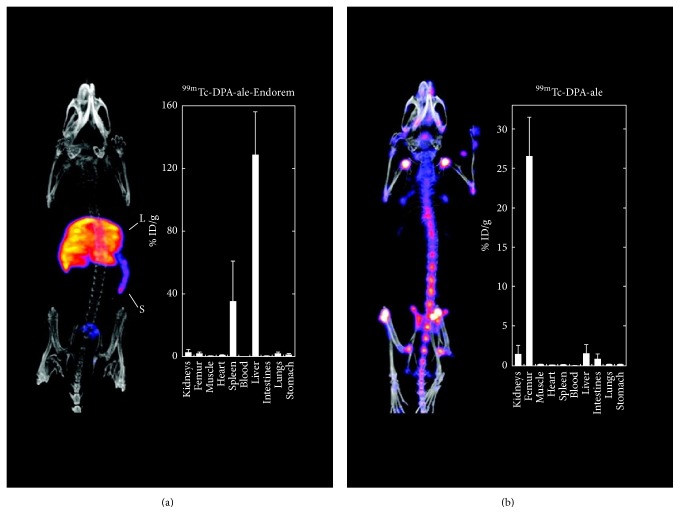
In vivo* SPECT/CT maximum intensity projection (left) and biodistribution studies (right) of (a) ^99m^Tc-DPA-alendronate-Endorem and (b) ^99m^Tc-DPA-alendronate. Reproduced, with permission, from [22].
